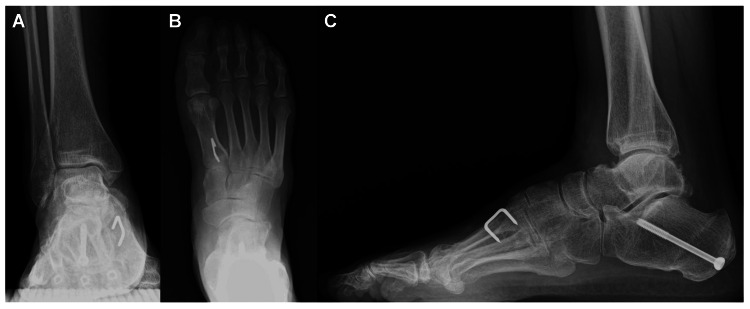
Plain radiographs on weight-bearing at 20 months after surgery. (A) Anteroposterior view of the ankle; (B) anteroposterior view of the foot; (C) lateral view of the foot.
